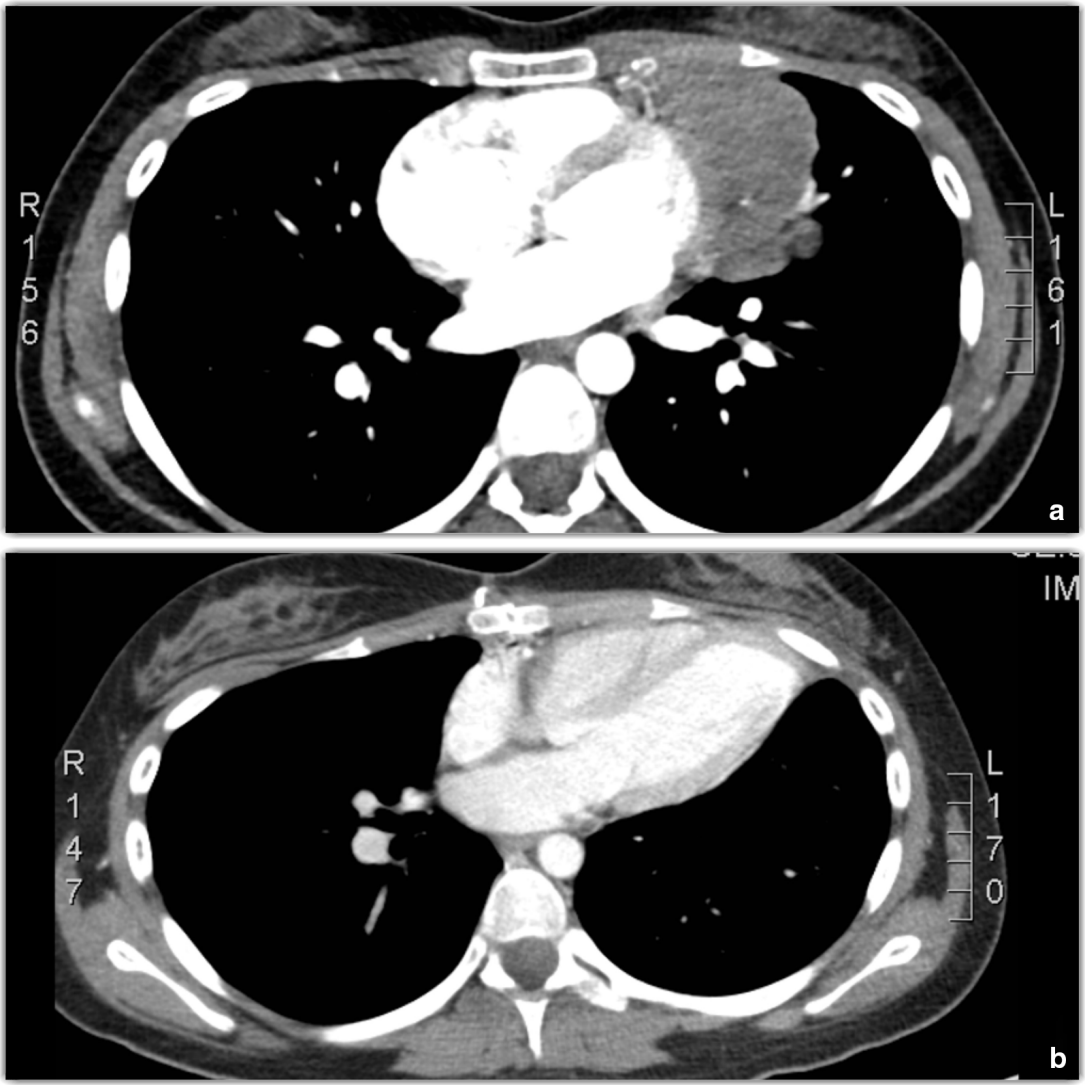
Chest computed tomography (CT) scan at baseline and after surgery. a Chest CT scan showing thymoma at baseline. b Postsurgical chest CT scan with no residual disease
